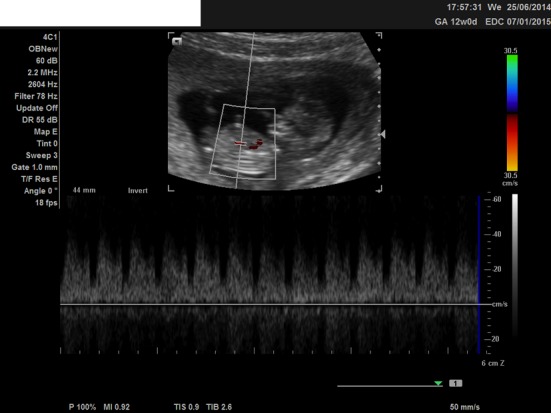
Ultrasound image of the first fetus at 13 weeks, showing the nuchal translucency.
